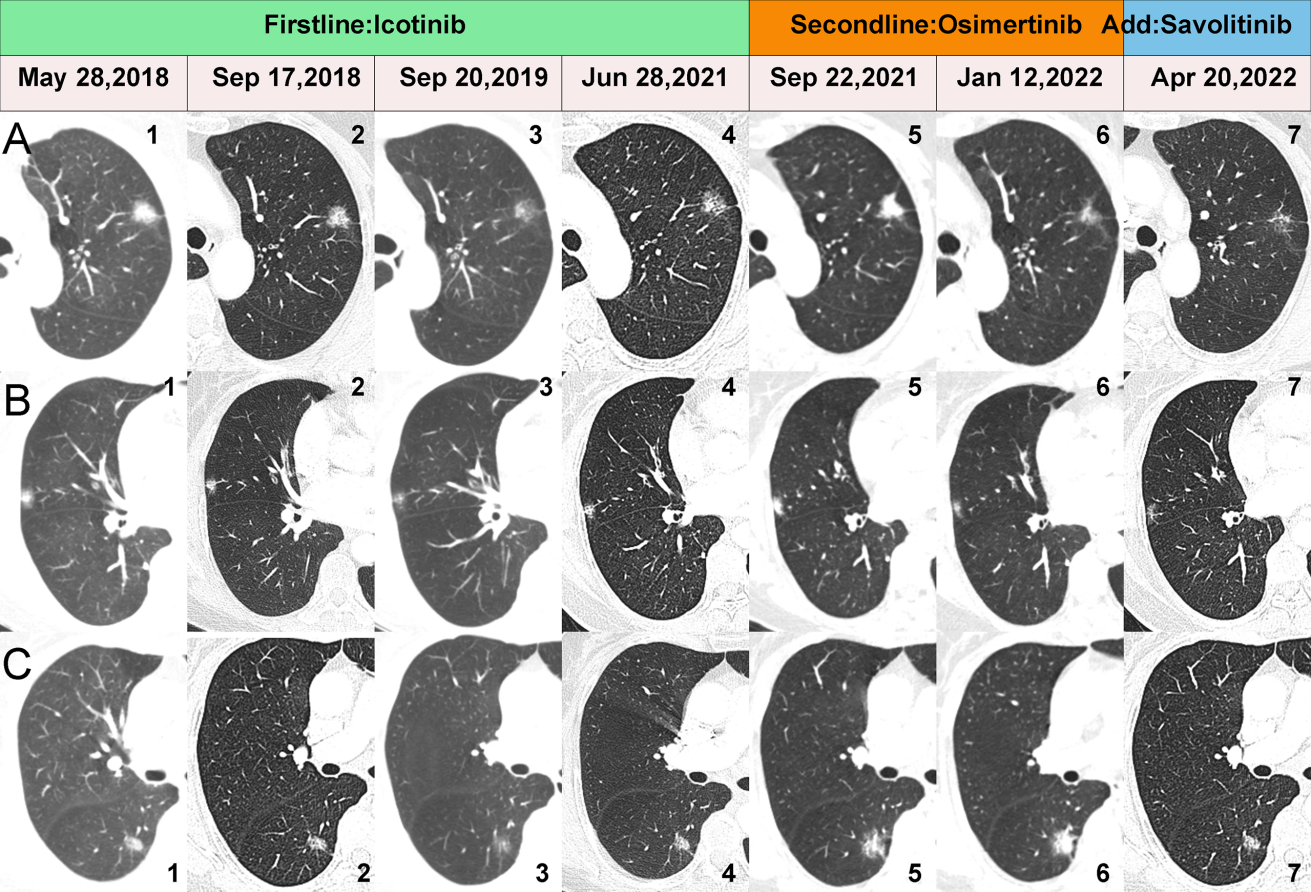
CT scans showing changes in the three lesions during the whole treatment. (A1-7) show the dynamic changes of the L1. (B1-7) show the dynamic changes of the L2. (C1-7) show the dynamic changes of the L3.
